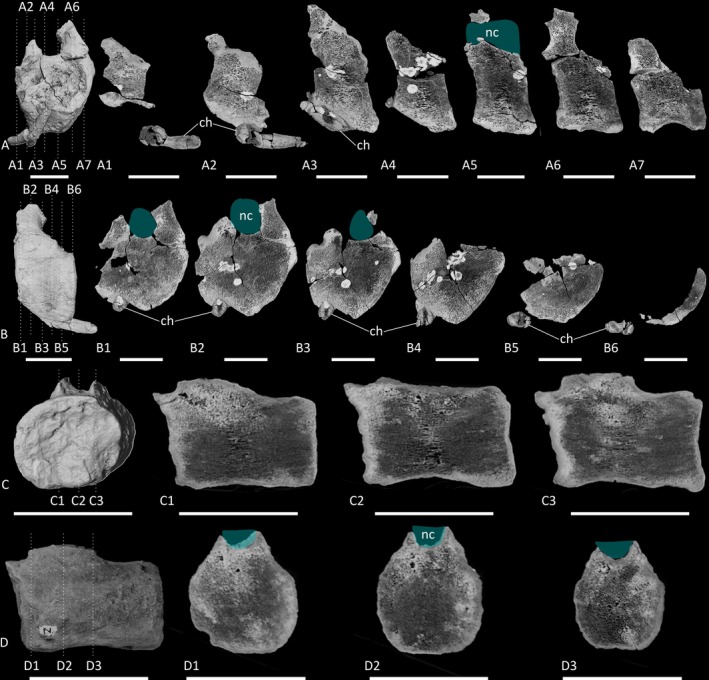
CT scan of *Wintonotitan wattsi* anterior and middle caudal vertebrae QMF7292. (A, C) 3D rendering anterior view, (B) 3D rendering left lateral view, (D) photograph left lateral view; (A, B) anterior caudal vertebra ‘E’, (C, D) middle caudal vertebra ‘N’; (A1–A7, C1–C3) CT scan slices in parasagittal cross‐section, (B1–B6, D1–D3) CT scan slices in transverse cross‐section. Ch, chevron; nc, neural canal. Key: Blue highlight, neural canal. Scale bars = 100 mm.
